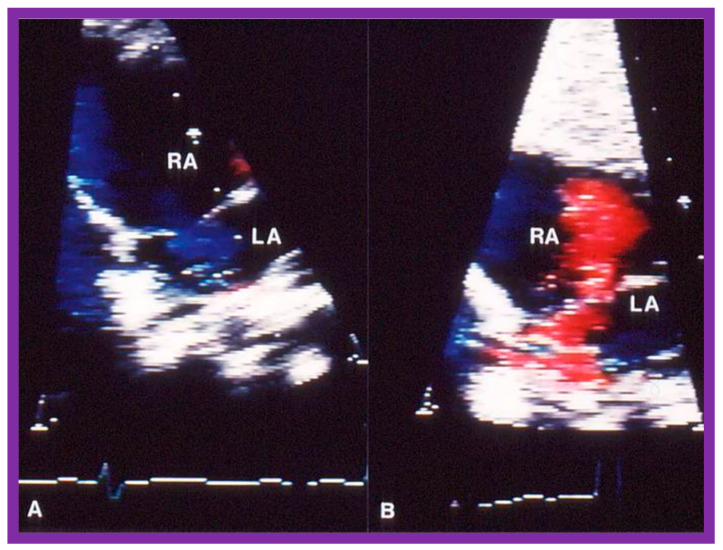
Selected video frames of the atrial septum, demonstrating a right-to-left shunt by color Doppler, across the patent foramen ovale prior to balloon pulmonary valvuloplasty (A) which has changed to a left-to-right shunt (B) 24 h later. LA, left atrium; RA, right atrium. Reproduced from Reference [75].
